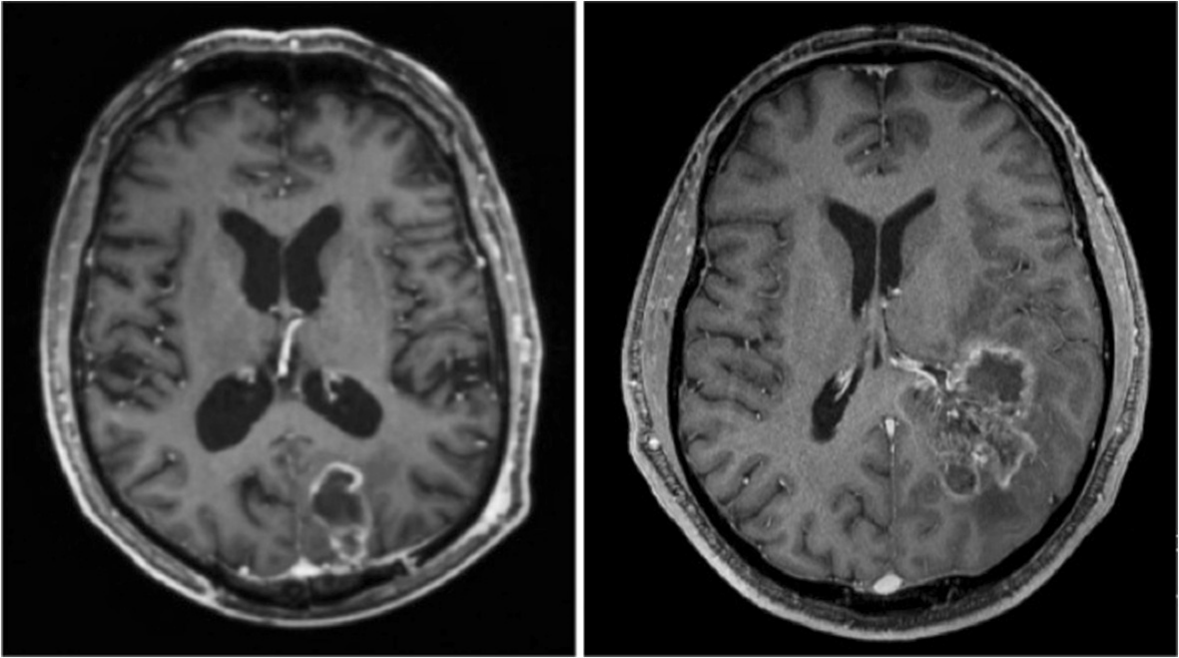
Glioblastoma on T1-weighted, post-contrast magnetic resonance images on the axial view. Tumor localization in the cortex without subventricular zone involvement (left). Glioblastoma with infiltration of the subventricular zone and subependymal spread (right).
